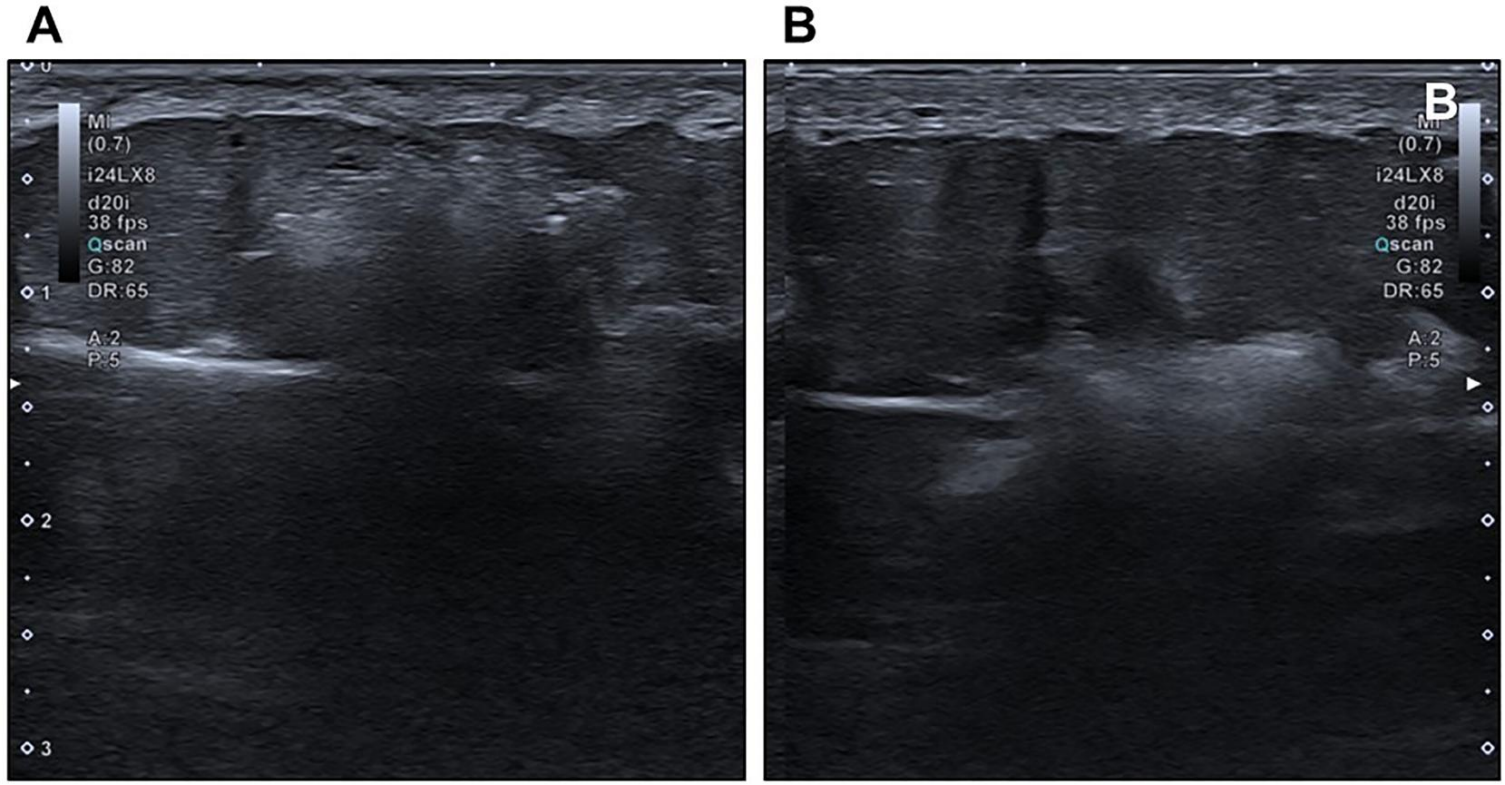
Demonstration of the ultrasonographic aspects of the Rennova Body Shape^®^ body HA. The following were observed: (a) Pure forms of HA; (b) Diluted forms of HA. The images were visualised with the aid of the Canon Aplio I800 device. HA, hyaluronic acid.
